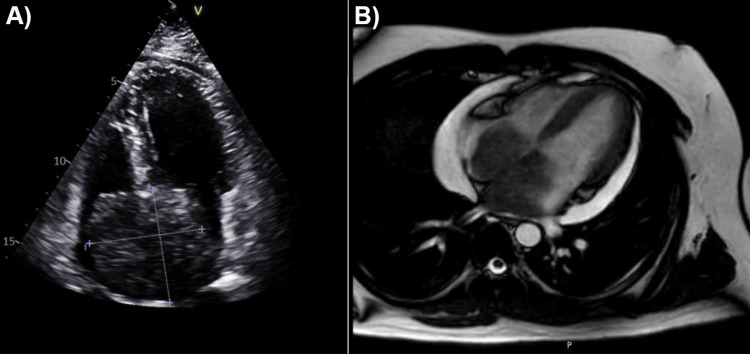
Cardiac imaging findings A) Transthoracic echocardiography: apical four-chamber view, demonstrating a large hyperechogenic intracardiac mass arising from the interatrial septum and extending into both atria. B) Cardiac magnetic resonance imaging: large biatrial mass with a moderate circumferential pericardial effusion
